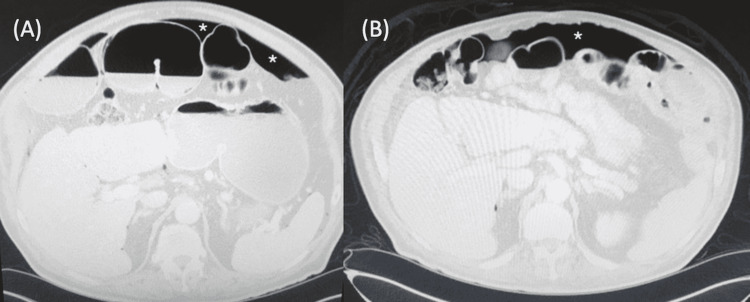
Pneumoperitoneum (asterisk) seen on CT from the patient’s first admission (A) and second admission (B).
